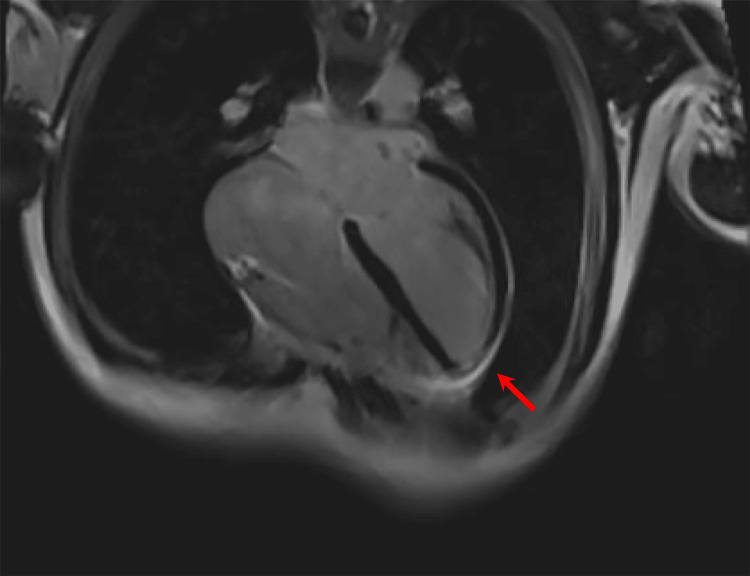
Axillary cardiac MRI view demonstrating diffuse pericardial enhancement (red arrow) consistent with a diagnosis of pericarditis.
